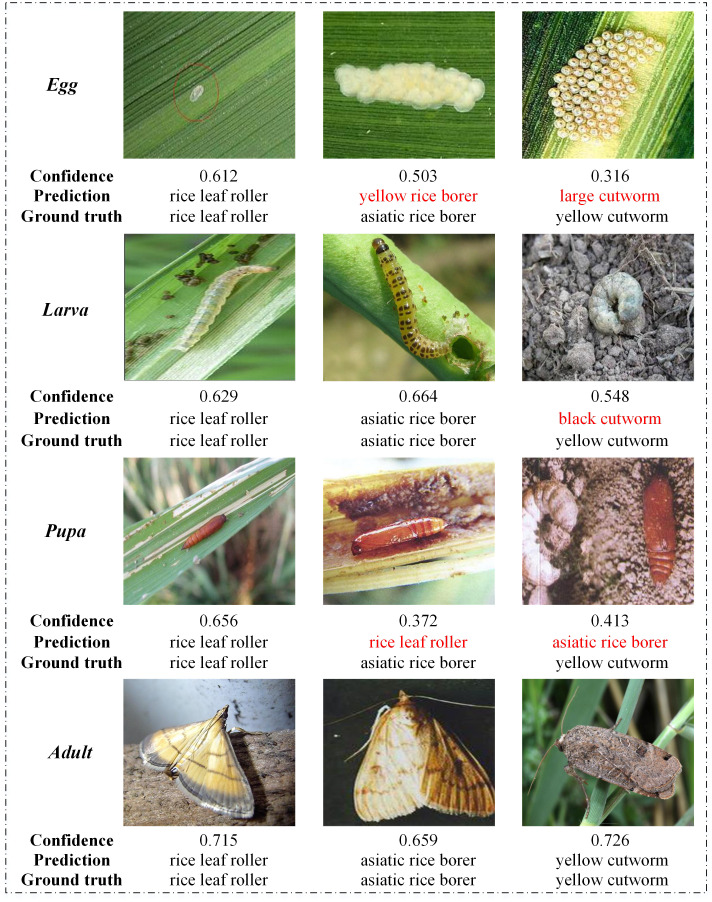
Prediction results of CT-PestNet for some samples from the IP102 dataset.
